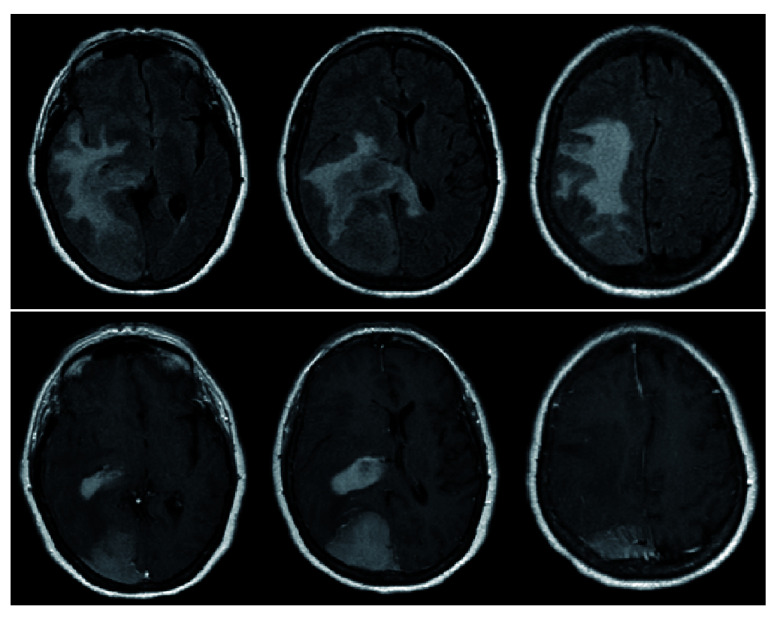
Pre-operative MRI demonstrating diffuse FLAIR changes with evidence of FLAIR signal crossing midline via the splenium of the corpus callosum (top). T1-post contrast reveals 2 distinct lesions – a homogenously enhancing extra-axial lesion in the right parietal lobe as well as a homogeneously enhancing periventricular lesion (bottom).
